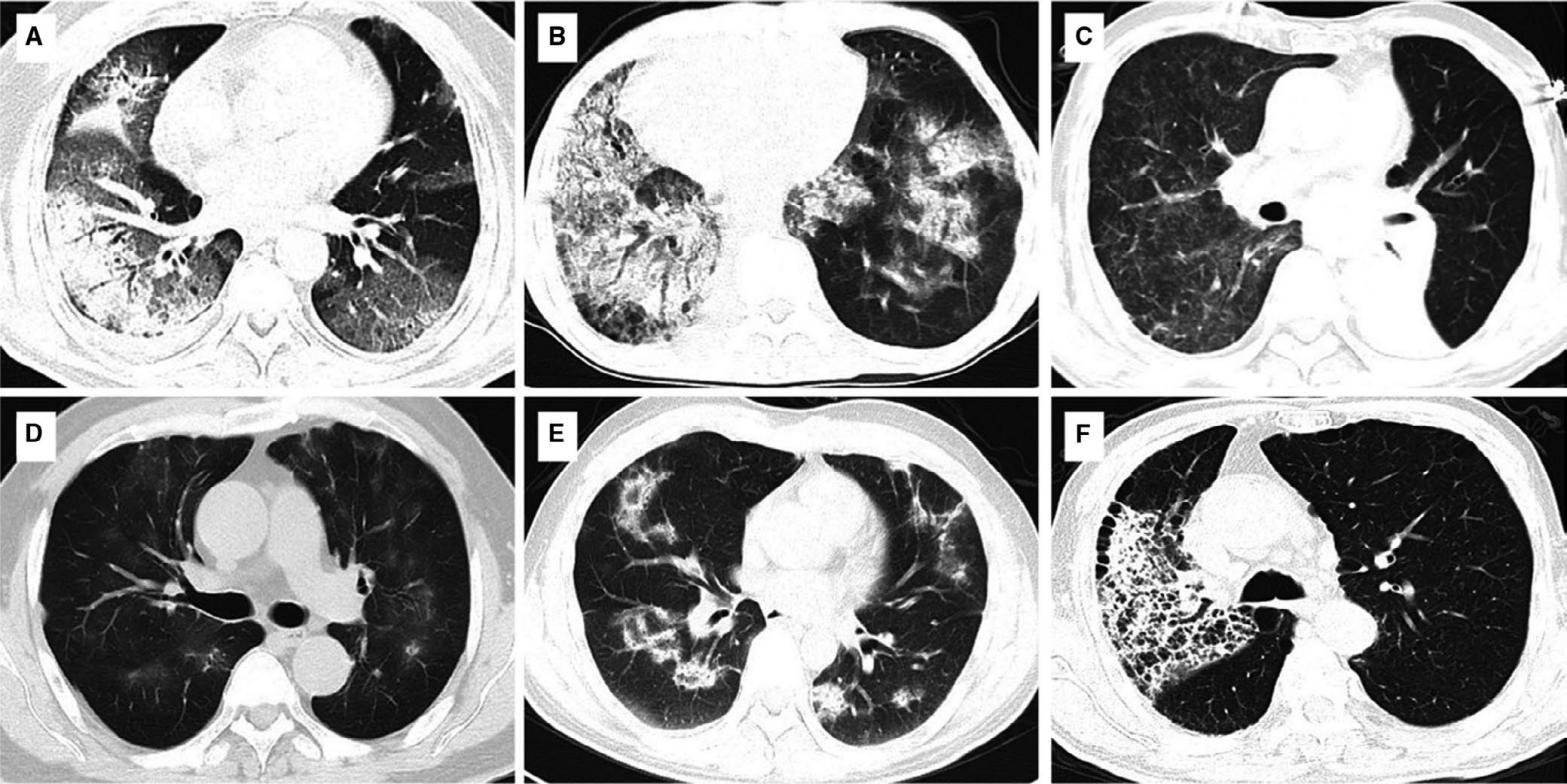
Representative radiological features of immune checkpoint inhibitor‐related pneumonitis. A and B, CT images showing diffuse alveolar damage or acute interstitial pneumonitis, the patients presented with explosive onset and rapid respiratory failure; C, unilateral ground glass opacity; D, multiple ground glass opacities distributed along the bronchovascular bundles, showing hypersensitivity pneumatism like changes; E, multiple consolidation shadows with anti‐halo signs distributed along the bronchovascular bundles, showing cop‐like changes; F, consolidations confined in one lobe of lung, with reticular shadows.
